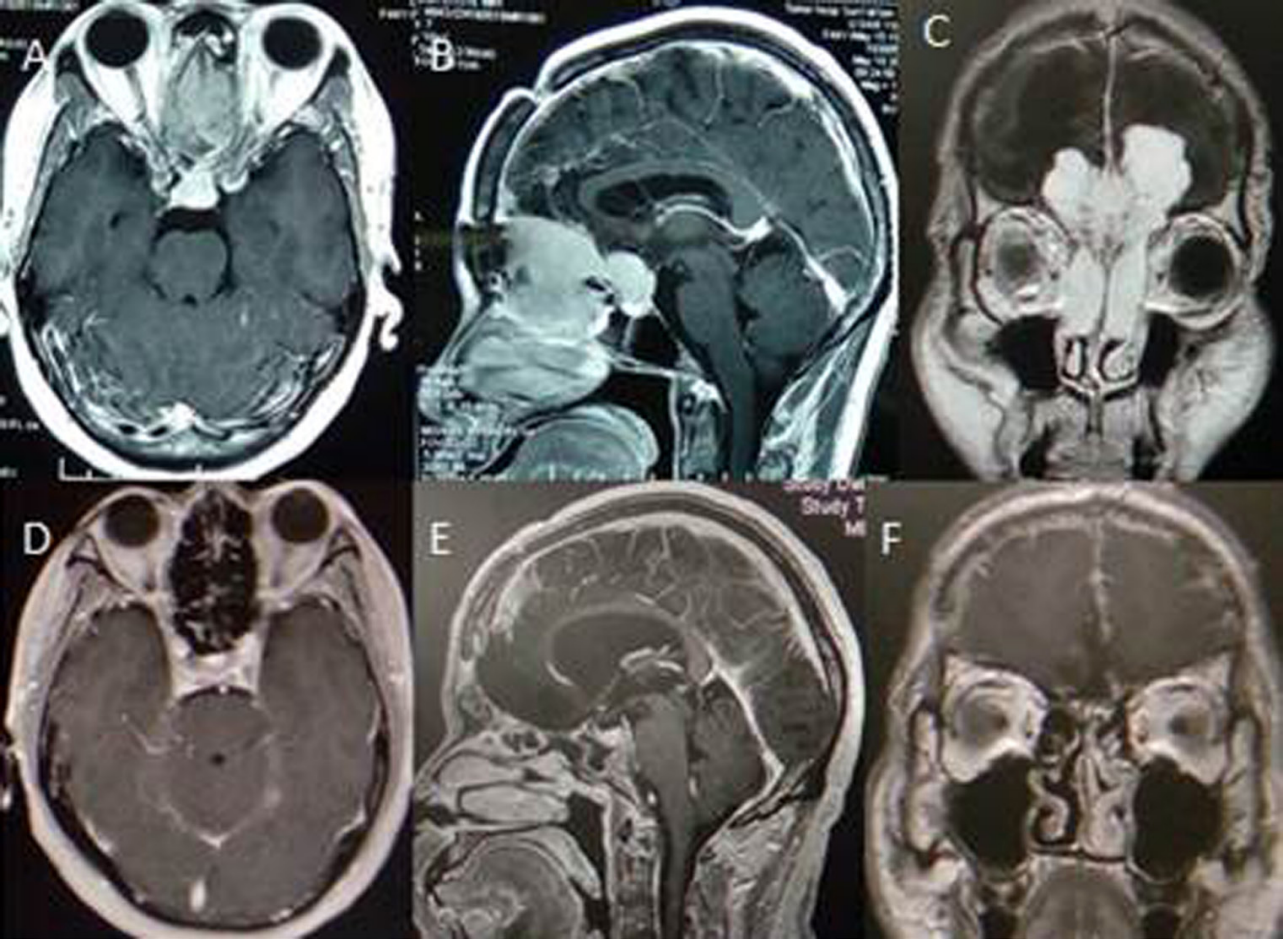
Cranial MR image displaying olfactory groove tumor with nasal cavity extension and follow-up with no recurrence
